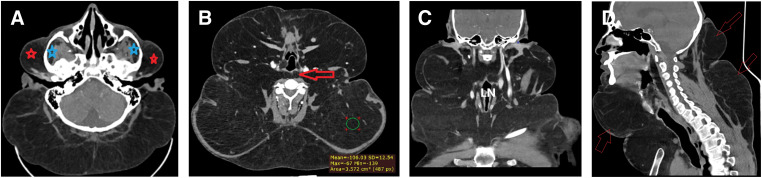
Neck CT scan. (A) Axial view at the level of the posterior fossa showing extensive non-encapsulated subcutaneous fat deposition extending bilaterally to parotid (red asterisks) and masticator spaces (blue asterisks). (B) Axial view at the level of the larynx showing fat deposition deep to sternocleidomastoid muscles bilaterally and in the prevertebral space (red arrow). (C) Post-contrast coronal view showing extensive non-encapsulated subcutaneous fat deposition. (D) Sagittal view showing anterior and posterior neck involvement with posterior “buffalo hump” appearance (red arrows).
